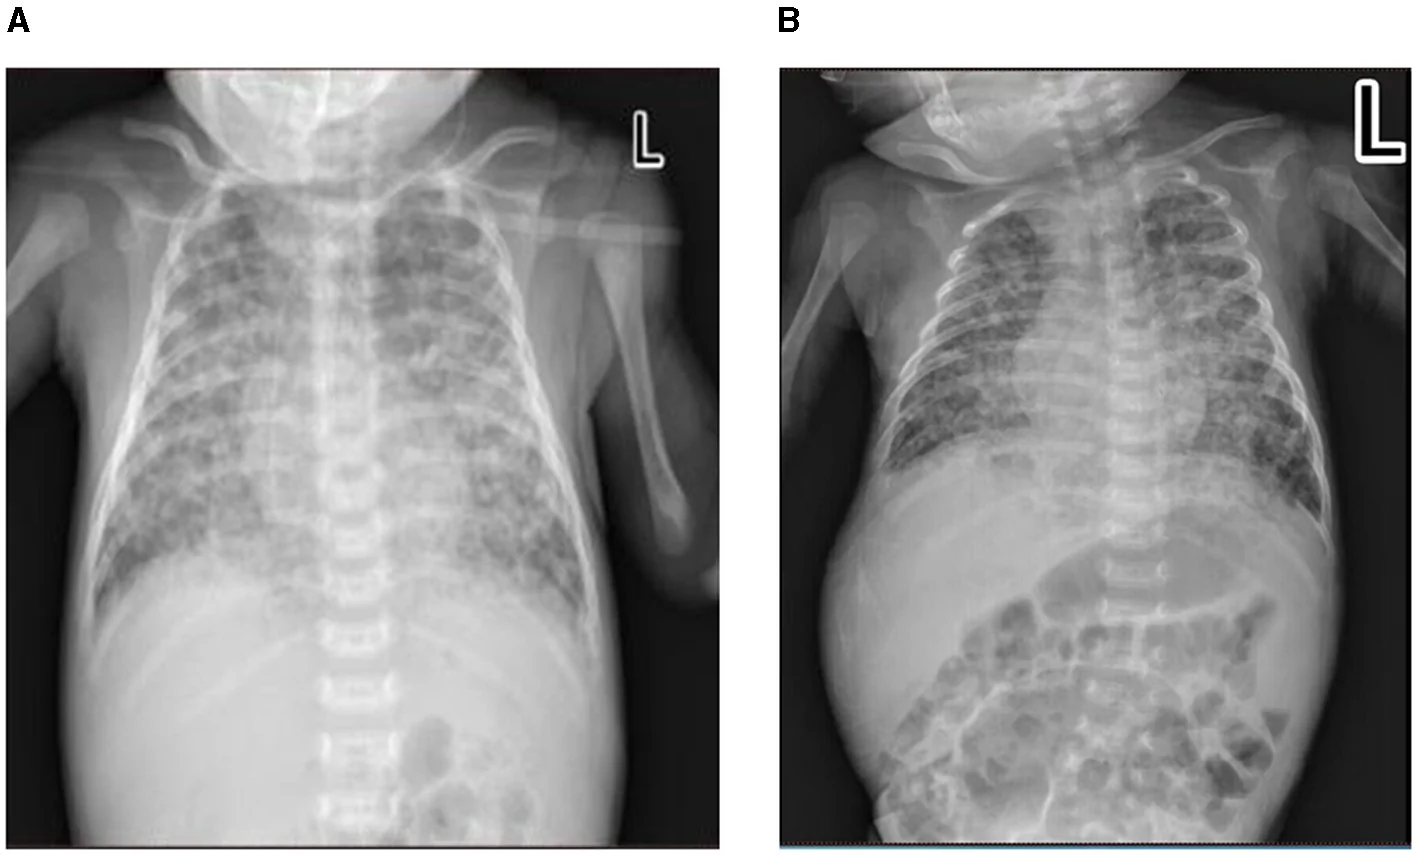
Chest X-ray of the patient at diagnosis of congenital tuberculosis (TB). (A) In the early stages of anti-TB treatment/day of life 25. (B) On the 26th day of anti-TB on day of life 47.
